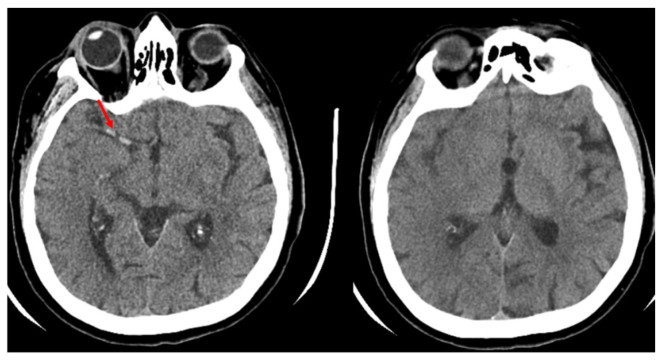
Patient case example: Evaluation of multimodal CT imaging. A 73-year-old patient was admitted with clinical signs of acute ischemic stroke. The initial NIHSS score was 21. The axial images of the non-enhanced head CT show a loss of grey-white matter differentiation in an extensive region within the territory of the right middle cerebral artery (MCA). The ASPECTS was 3 points. In addition, a thrombus is visible in the proximal segment of the right MCA, indicated by the hyperdense artery sign (marked with an arrow).
